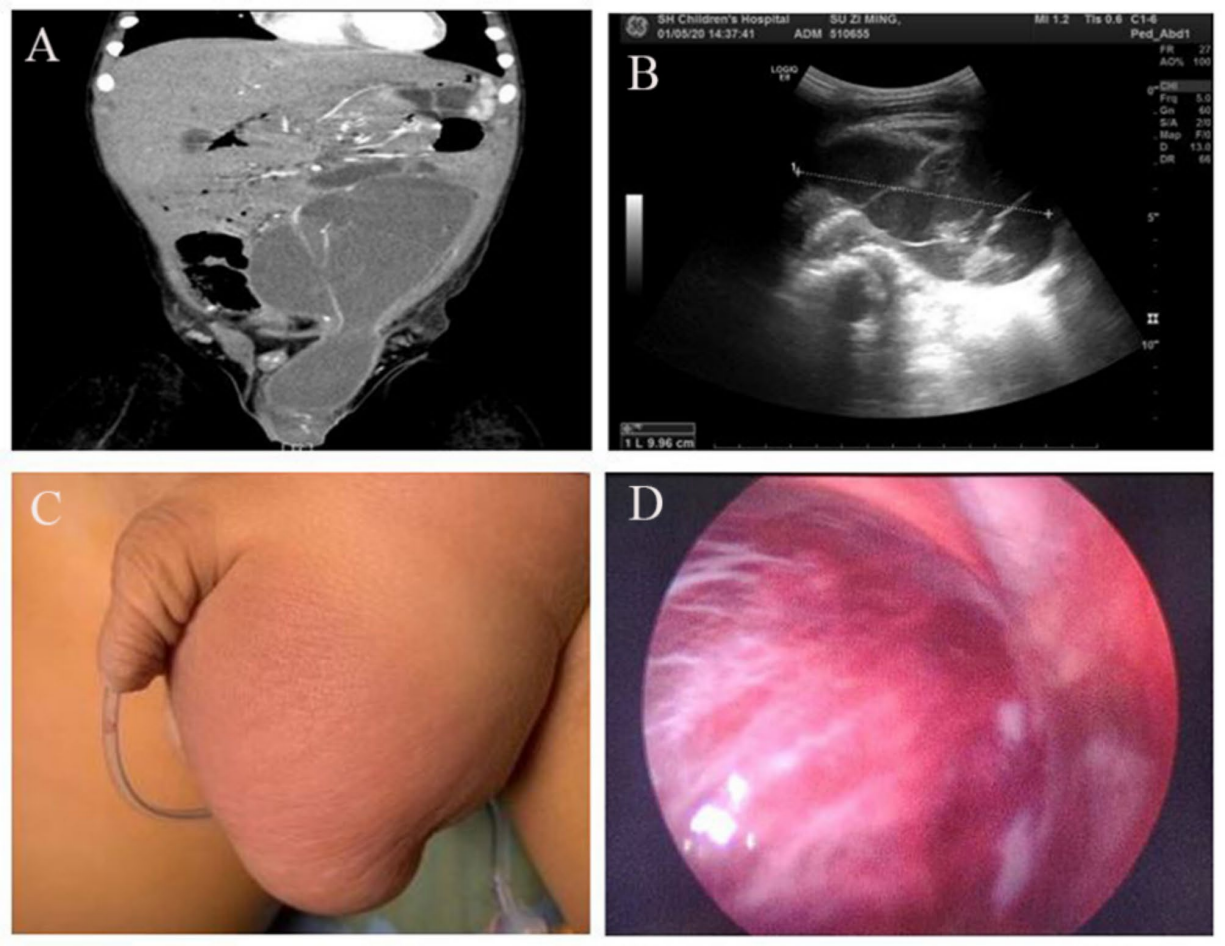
Patient (Case 8) misdiagnosed with an incarcerated inguinal hernia. A: Contrast-enhanced computed tomography scan; B: ultrasound image. C: The patient had a red, swollen, and painful mass measuring 4 × 5 × 4 cm in the left inguinal region before surgery. D: Surgical image
